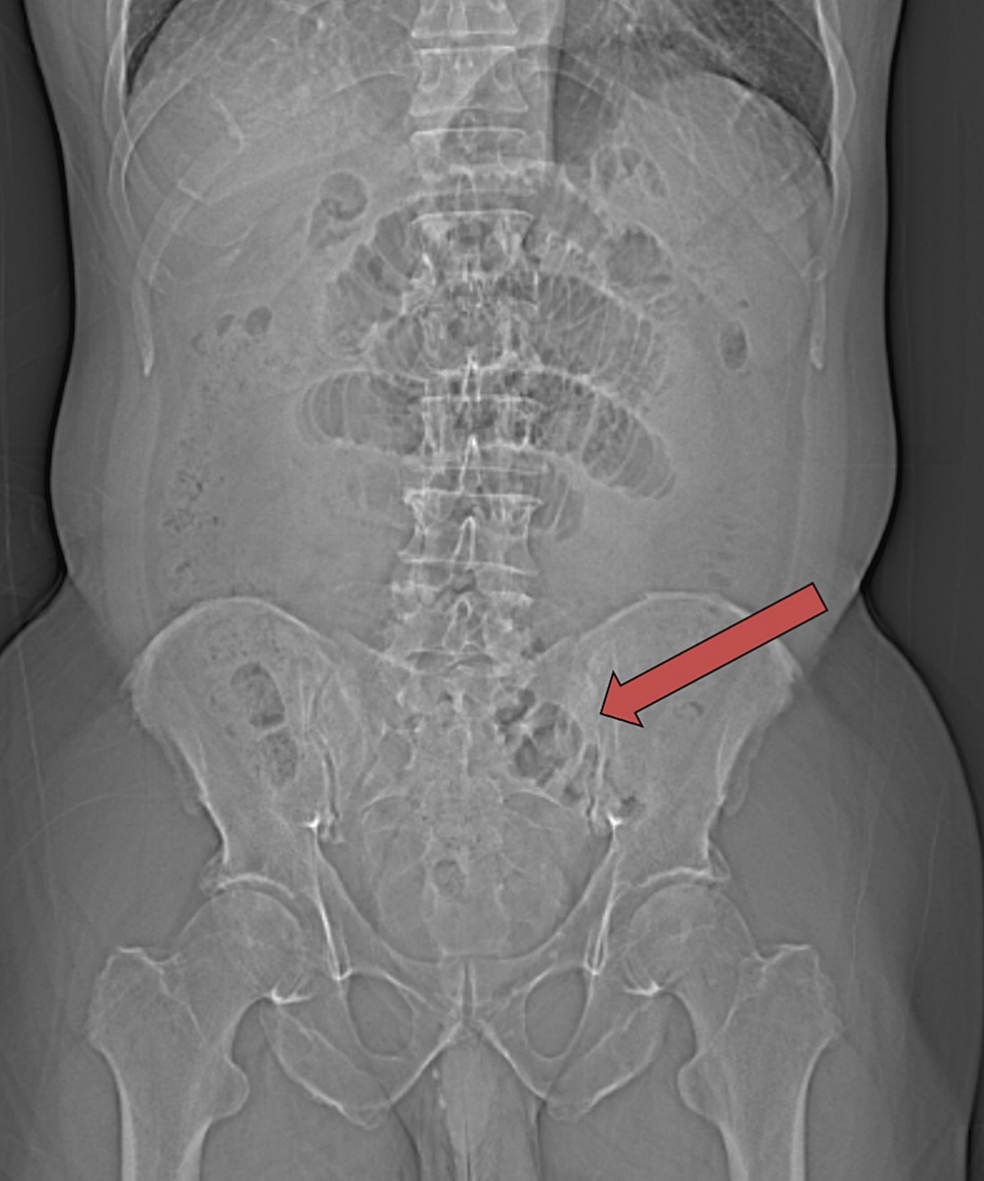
Anterior-posterior X-ray of the abdomen with arrow pointing to one of the many air-fluid levels found preoperatively.
